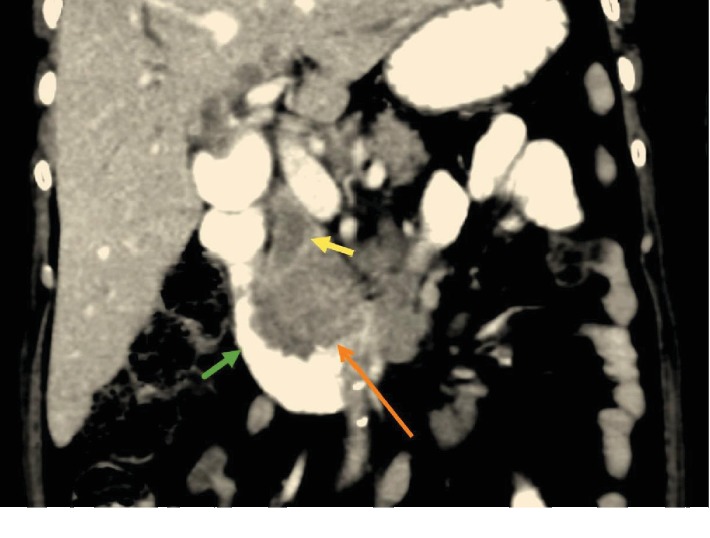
Enhanced computer tomography (CT) of the abdomen. Coronal slice obtained through the second part of the duodenum (D2; green arrow) shows an uncinate process mass (orange arrow) protruding into the lumen of D2, infiltrating and obstructing the dilated distal common bile duct (yellow arrow).
